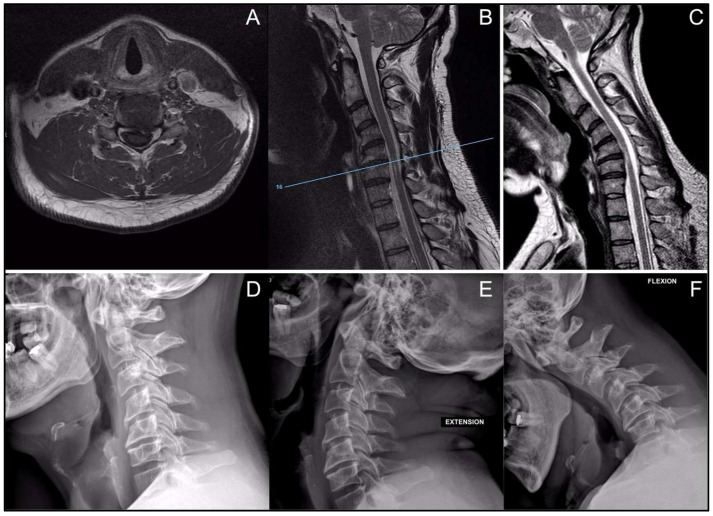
(A) Axial T1-weighted MRI at C5–C6 showing a small left-sided osteophyte. (B) Sagittal T2-weighted MRI demonstrating intramedullary hyperintensity (myelomalacia); axial level reference marker shown. (C) Dynamic sagittal T2-weighted MRI in maximal flexion demonstrating anterior cord–osteophyte contact at C5–C6; the T2 hyperintensity is located more caudally on neutral imaging, with flexion revealing the conflict. (D–F) Lateral cervical radiographs: neutral (D), extension (E), and flexion (F).
